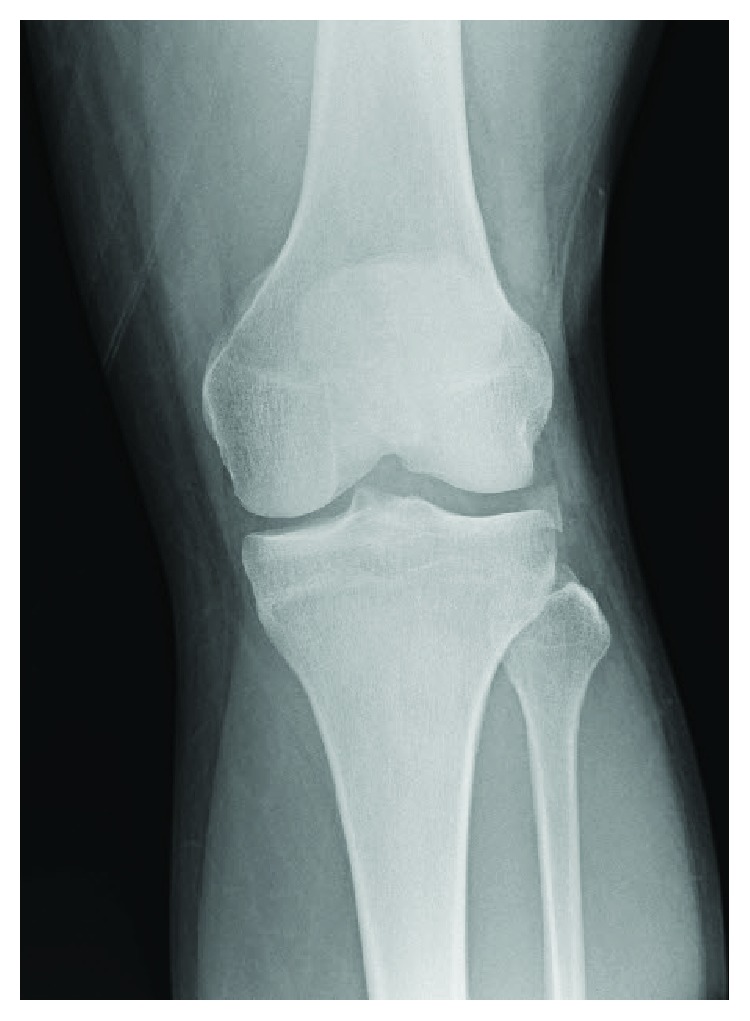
An anteroposterior radiograph of the patient's left knee demonstrating a Segond fracture.
